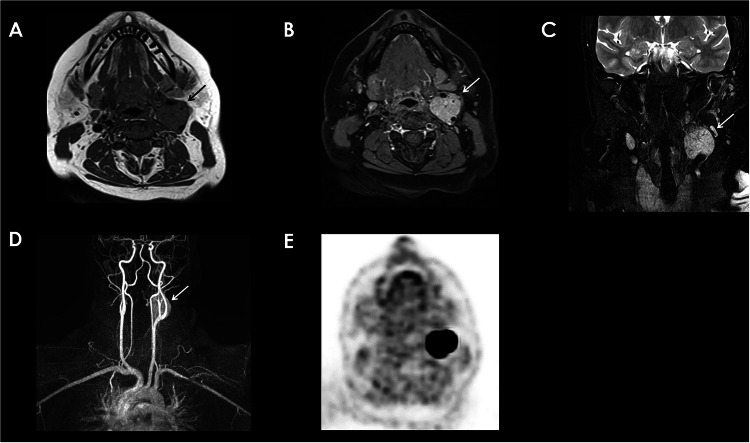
Left Carotid Body PGL. MRI (A to D): Well-circumscribed mass of intermediate signal intensity on Axial T1-w MR (A), high signal intensity on Coronal T2 MRI (C) was seen on the carotid bifurcation. It splays apart the internal (white dashed arrow) and external carotid (white arrow) arteries and expands the carotid bifurcation (B–D) “Lyre Sign”. The jugular vein is displaced posteriorly (B). It shows a “salt and pepper” appearance representing a combination of hemorrhagic regions/slow flow “salt” and regions of flow voids “pepper” (best seen on T2). After gadolinium administration, there is a vivid enhancement (B). Tricks® MRA sequence revealing the location of the PGL (D). High 68Ga-DOTA-SSTR uptake at PET-CT (E) SUVmax 58,8 (E)
